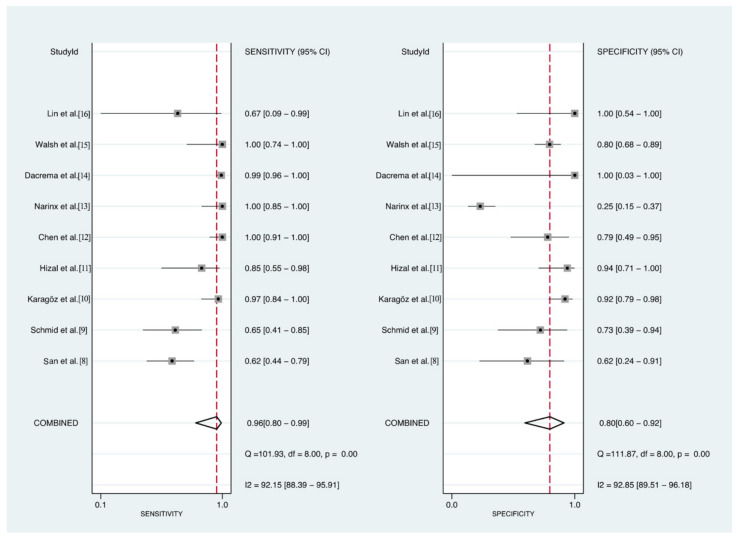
Positive (left panel) and negative (right panel) percent agreement of lung ultrasound for the diagnosis of interstitial pneumonia caused by COVID-19.
